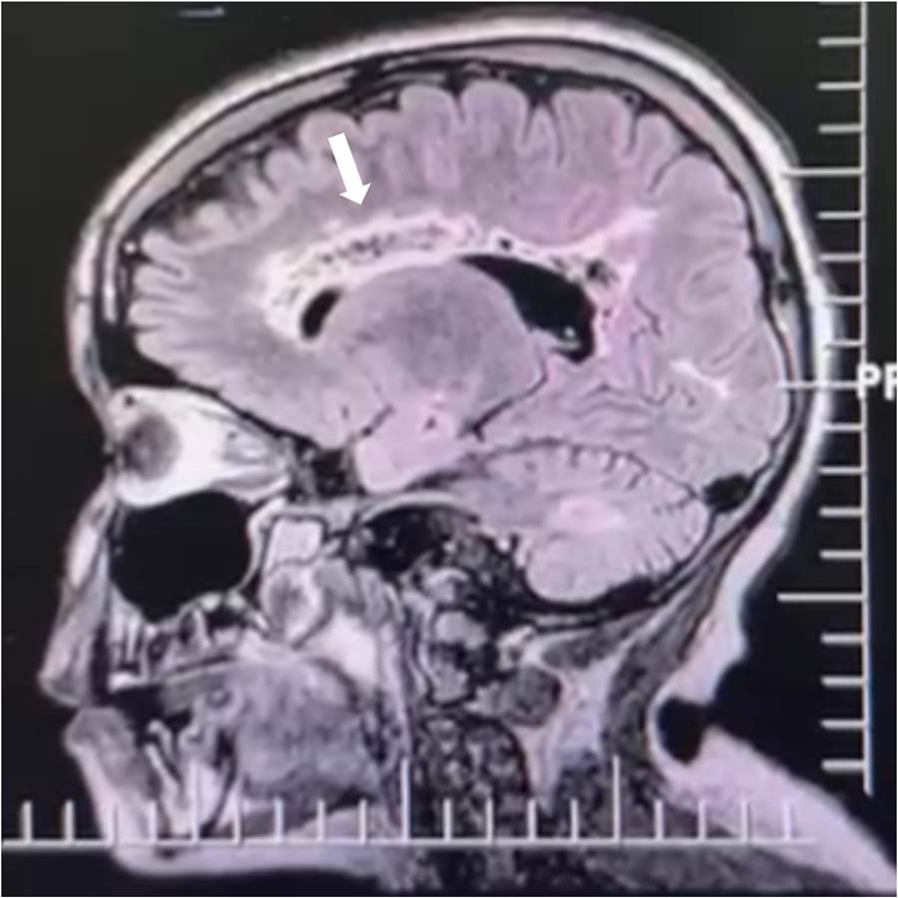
Magnetic resonance imaging (MRI) of the skull shows lesions with hyperintense signal, affecting the periventricular white matter of the corpus callosum up to the occipital lobe, sparing the temporal lobes and posterior fossa symmetrically.
